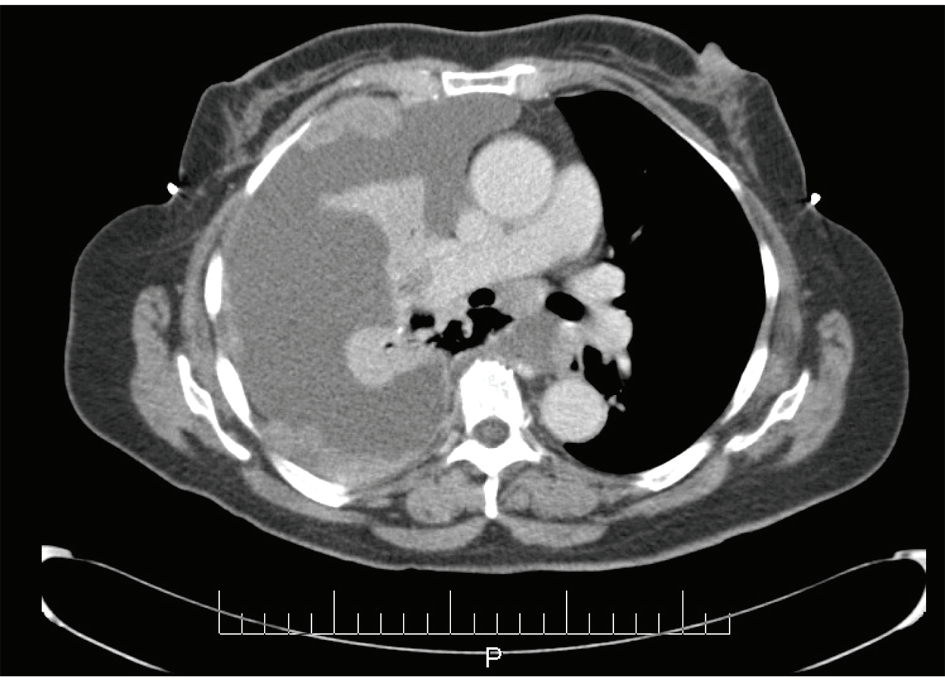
CT scan image demonstrating pleural effusion and nodularity.
